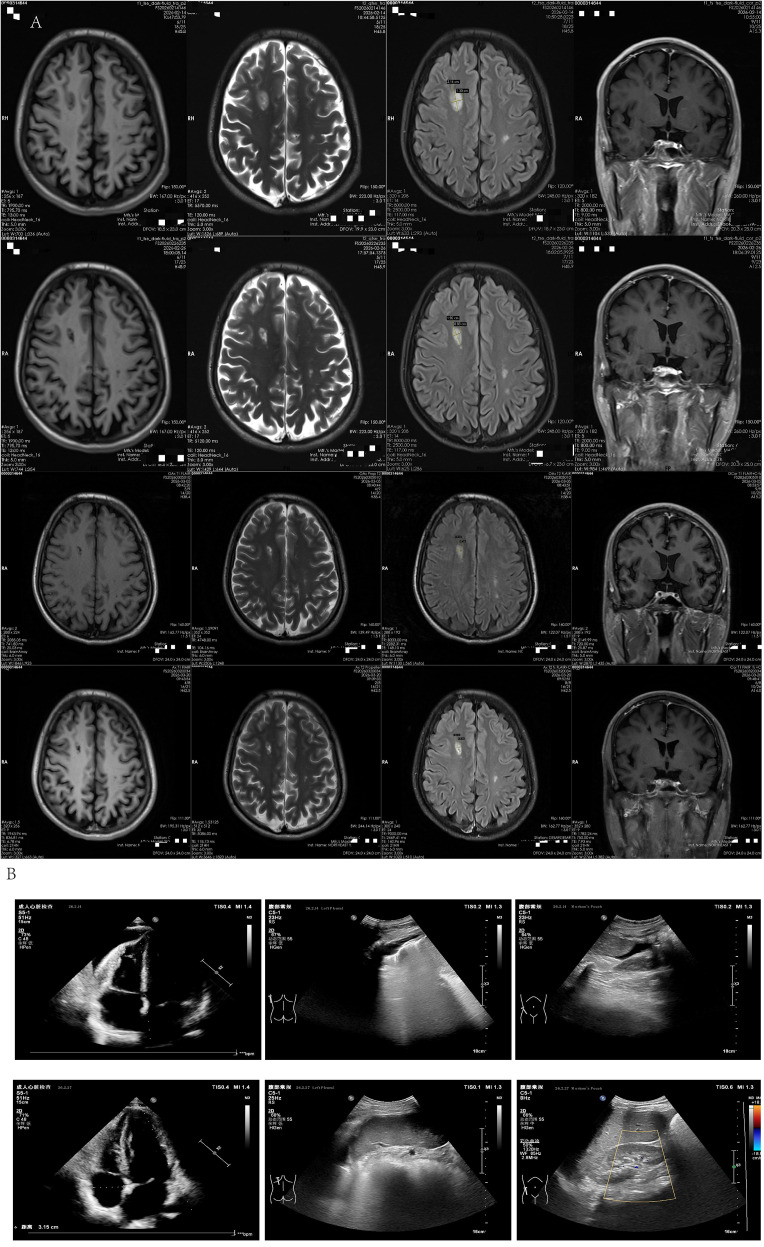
Serial imaging changes in lupus cerebritis and polyserositis. (A) Brain MRI images are arranged chronologically from top to bottom: February 14, February 27, March 5, and March 20. Lesion size is reported in centimeters (cm). (B) Ultrasonographic images show pericardial, pleural, and abdominal effusions before and after optimized LPE. MRI, magnetic resonance imaging; CNS, central nervous system; LPE, lymphoplasmapheresis.
